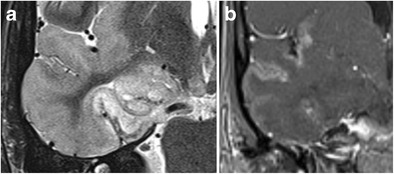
Herpes encephalitis. On coronal T2-weighted images (a) extensive corticosubcortical oedema is seen in the right temporal and insular lobe. Notice the presence of cortical T2-hypointense abnormalities in the right hippocampus and along the right collateral sulcus, denoting small petechial haemorrhages. On contrast, enhanced T1-weighted images (b), there is extensive right temporal and insular gyral enhancement
